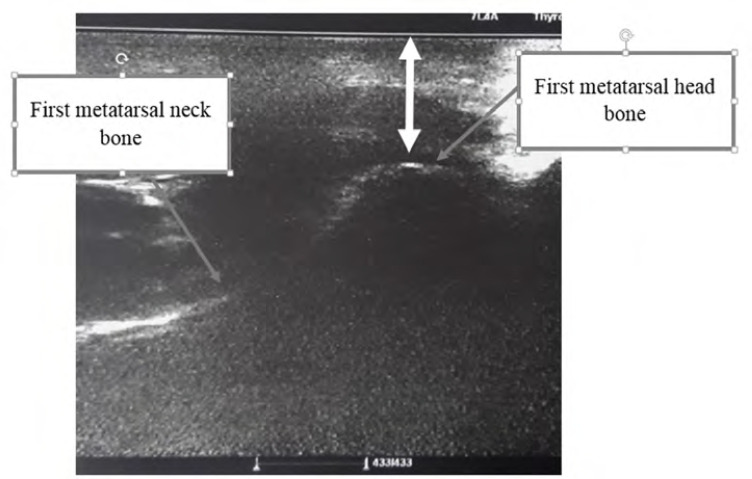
Measurement of first metatarsal head soft tissue thickness with ultrasound.
